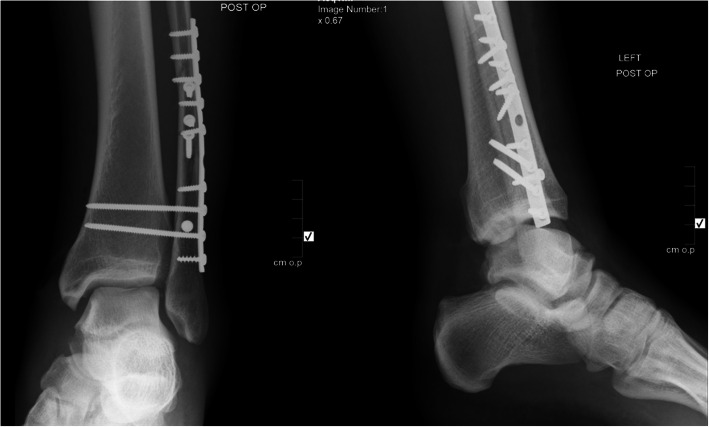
Post-operative anteroposterior and lateral plain ankle radiographs. Osteothynseis with lateral malleolus plate and two syndesmotic quadritcortical screws
